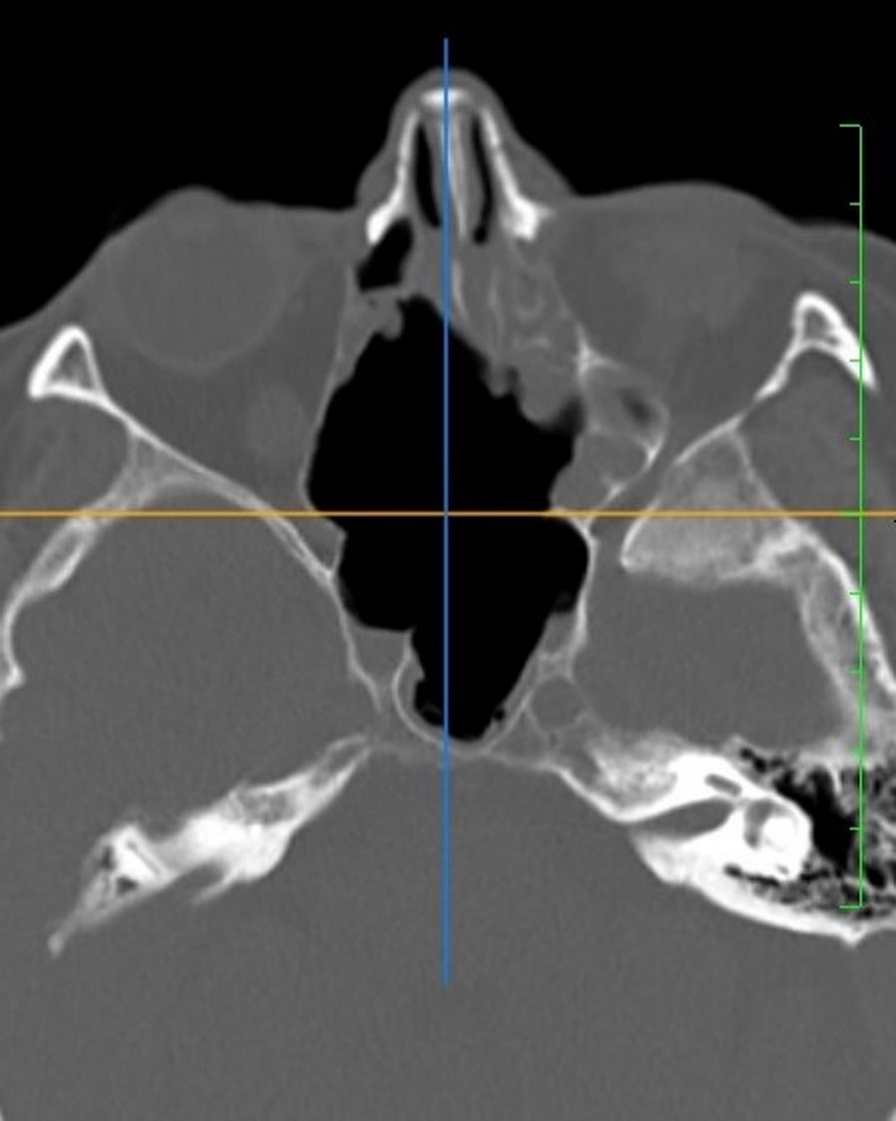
CT. Axial projection. State after radical resection of tumor masses—lack of the right superior and medial turbinate, medial wall of the right maxillary sinus, frontal walls of the sphenoid sinuses. Bare bone of the posterior part of the medial orbital wall, as mentioned in the text. The ethmoids that were well pneumatized before the surgical procedure are now filled with postoperative edematous tissues
